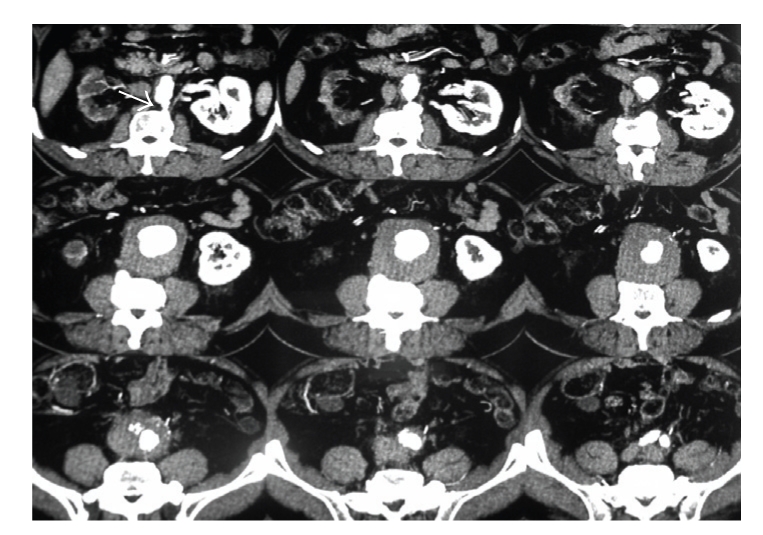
Cross-sectional CT scan demonstrates large abdominal aortic aneurysm compressing Inferior Vena Cava (IVC). The arrow points to juxta-renal IVC, the arrow head points to the virtually occluded IVC, and the line to the thrombosed common iliac veins.
